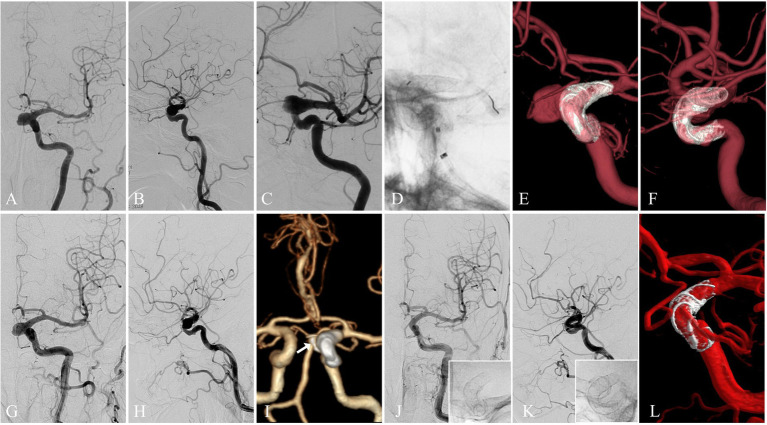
Angiographic images of a 69-year-old woman with a wide-neck aneurysm in the left ophthalmic ICA incidentally found after dizziness. (A) A-P and (B) lateral view of an aneurysm in the left ophthalmic ICA. (C) Intraprocedural working view of the aneurysm after TPFD (5 × 25 mm) deployment. (D) Fluoroscopic image of the TPFD. (E) Distal and (F) proximal apposition of TPFD confirmed by dual-volume 3D reconstruction. (G) A-P and (H) lateral view of incomplete occlusion of the aneurysm at the 6-month FU. (I) CTA image of the residual neck (arrowhead) of the aneurysm at the 12-month FU. (J) A-P and (K) lateral view of complete occlusion of the aneurysm at the 28-month FU. (L) Dual-volume 3D reconstruction at the 28-month FU.
